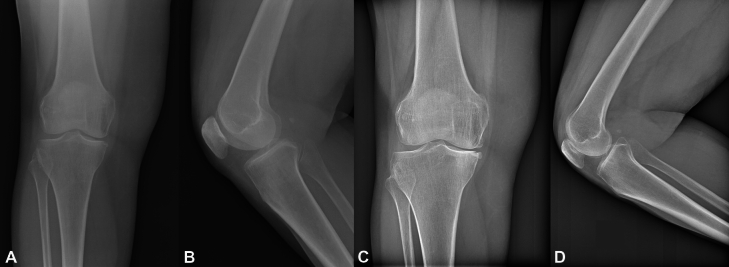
X-ray evaluation at baseline and at 4 years of follow-up after MFAT treatment in a 61-year-old woman with predominant medial knee OA. Minimal joint space narrowing, small osteophytes formation and subchondral sclerosis was found in the absence of clinical evolution at 4-year follow-up
